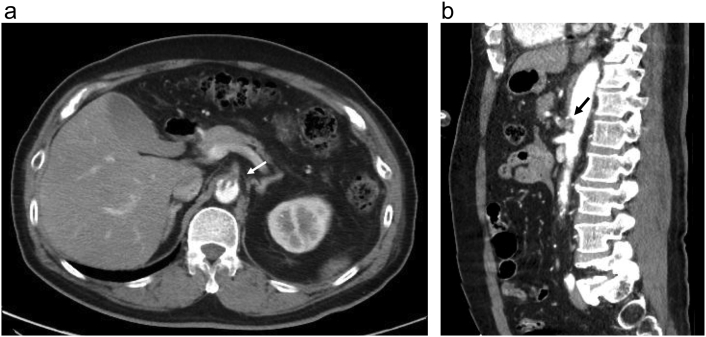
Axial (a) and sagittal (b) contrast-enhanced computed tomography images showing an intimal tear near the celiac artery origin (arrow).
